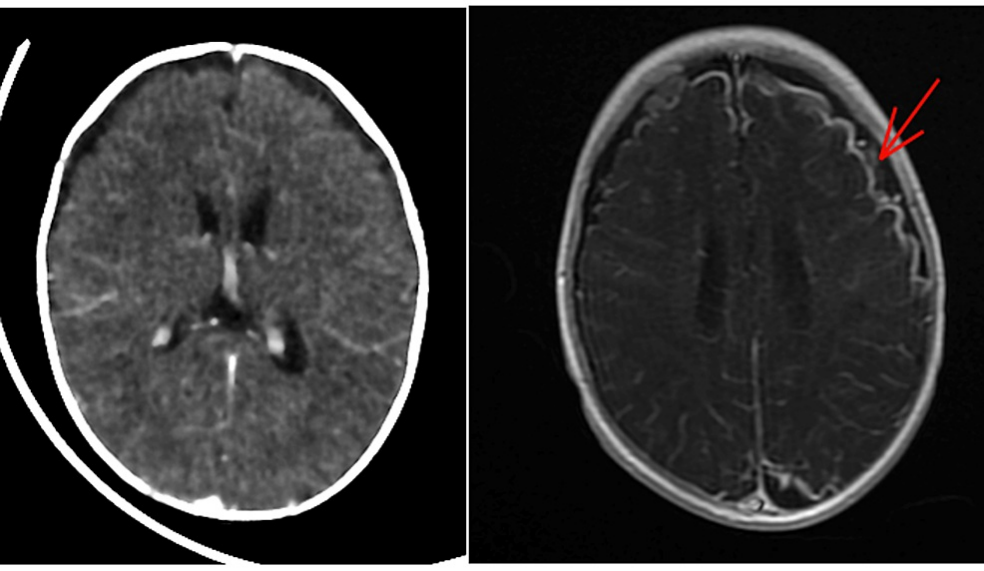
MRI of the brain Brain MRI showed leptomeningeal enhancement and minimal quadri-ventricular hydrocephalus (red arrow).
